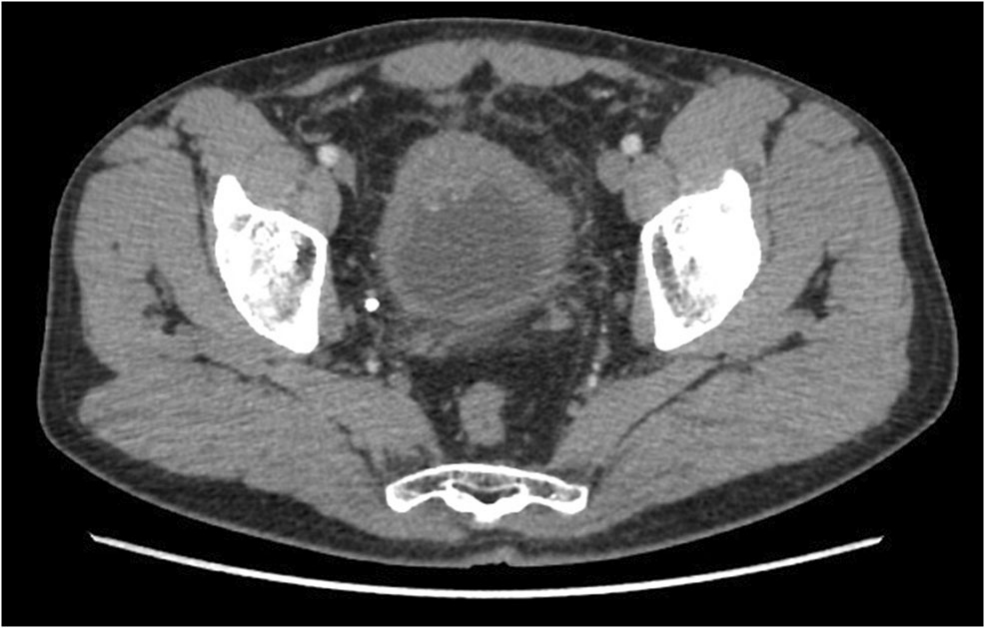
Contrast-enhanced computerized tomography (CECT) of the bladder: Contrast-enhanced computed tomography revealed a 46-mm lesion on the dome of the bladder, with concomitant thickening of the bladder walls
